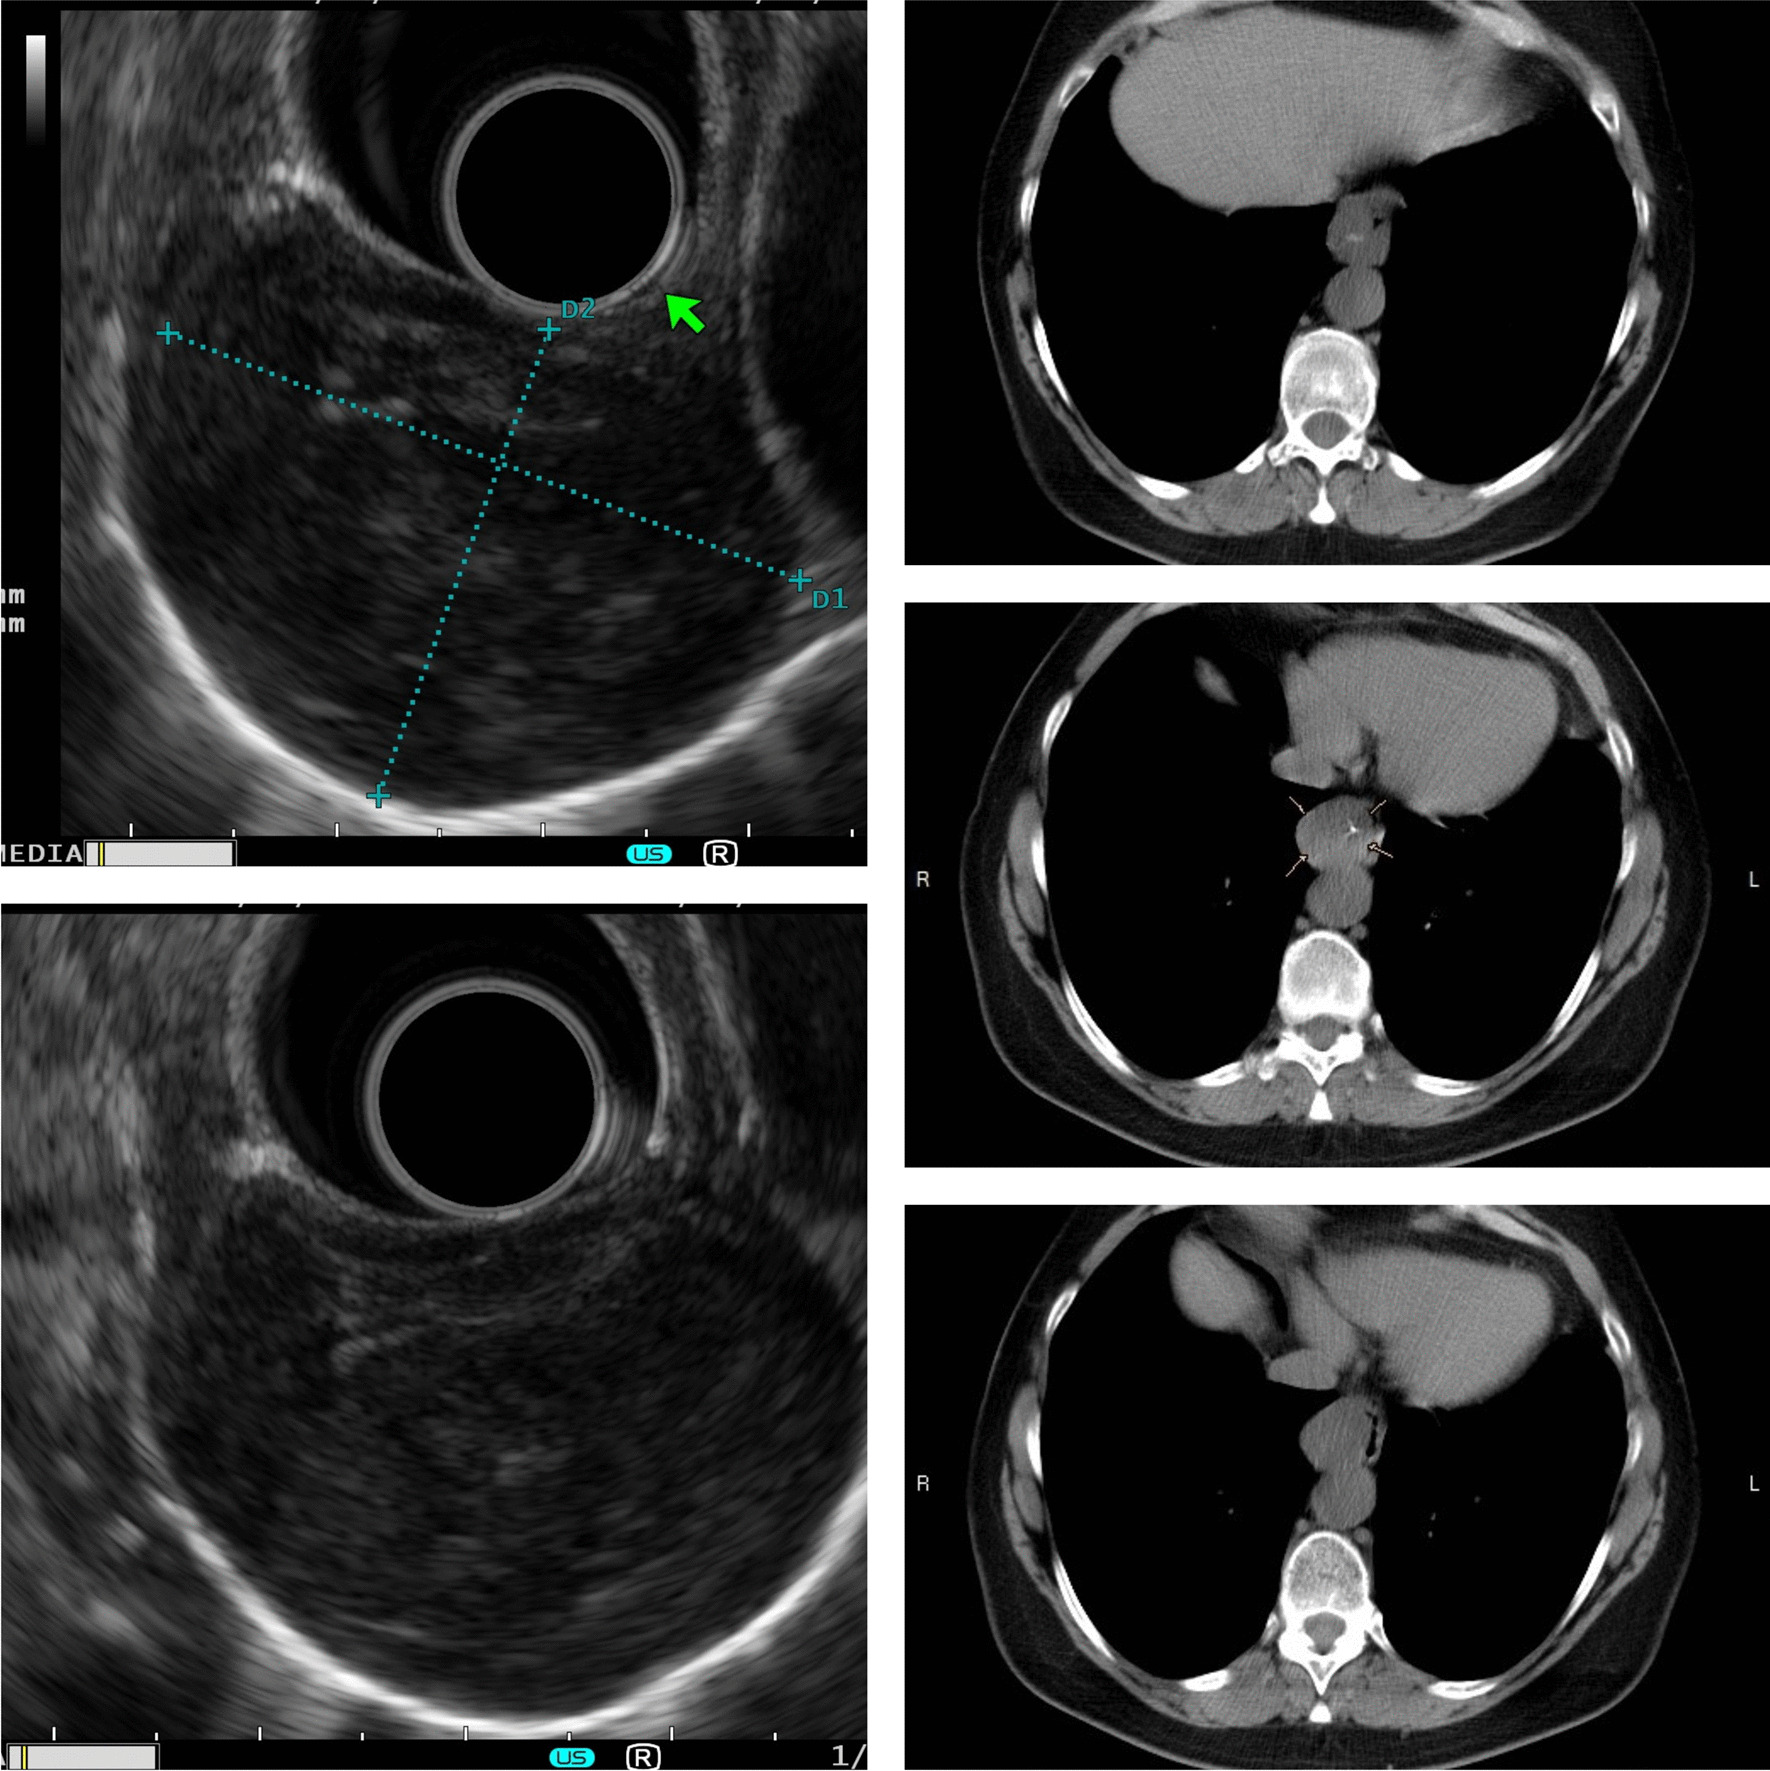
Computed tomography and endoscopic ultrasonography demonstrating wall thickening of the oesophagus causing stenotisation; photos were grouped together
